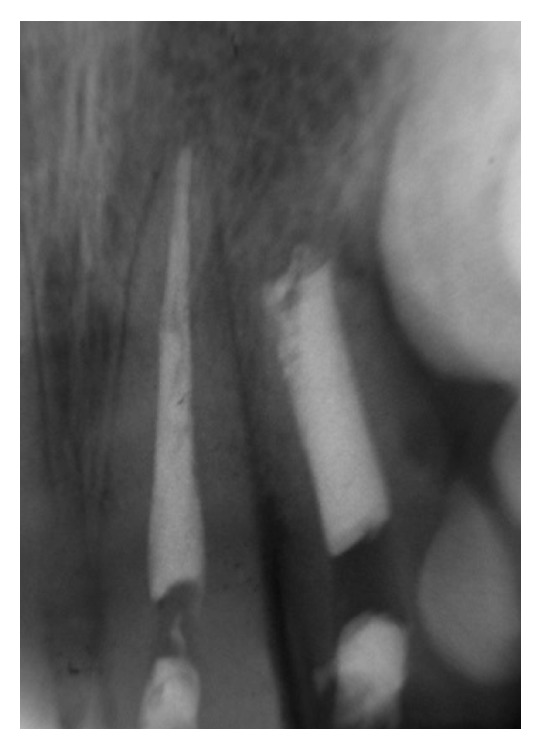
Periapical radiograph: root canal treatment finished in tooth 22 and tooth 21 in good condition after 1 year (seventh session; March 1999).
